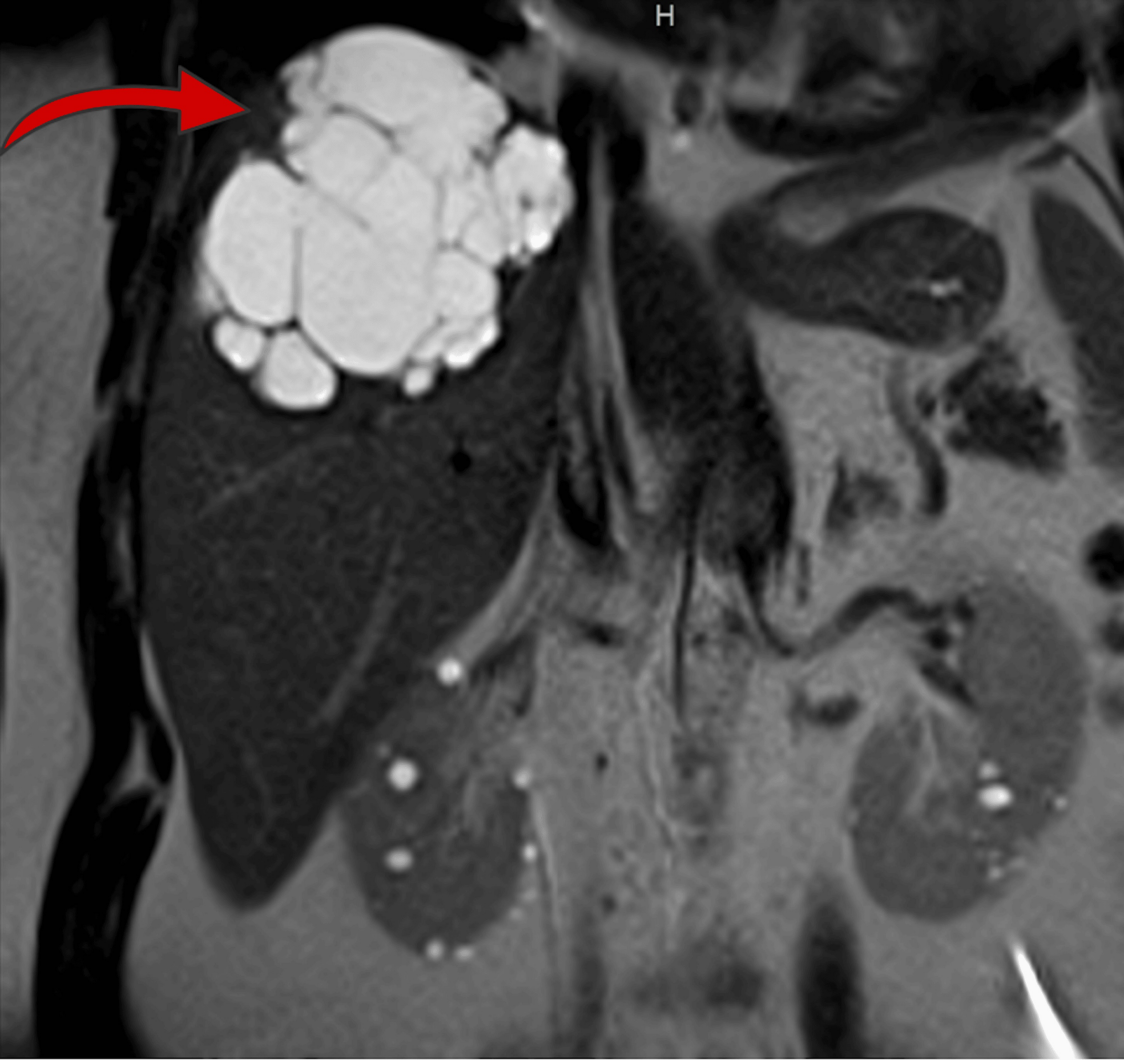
Magnetic resonance imaging (MRI) of the abdomen (coronal view) Coronal MRI image showing multiloculated cystic lesion measuring 8 × 9.4 × 9 cm involving hepatic segments VII and VIII
